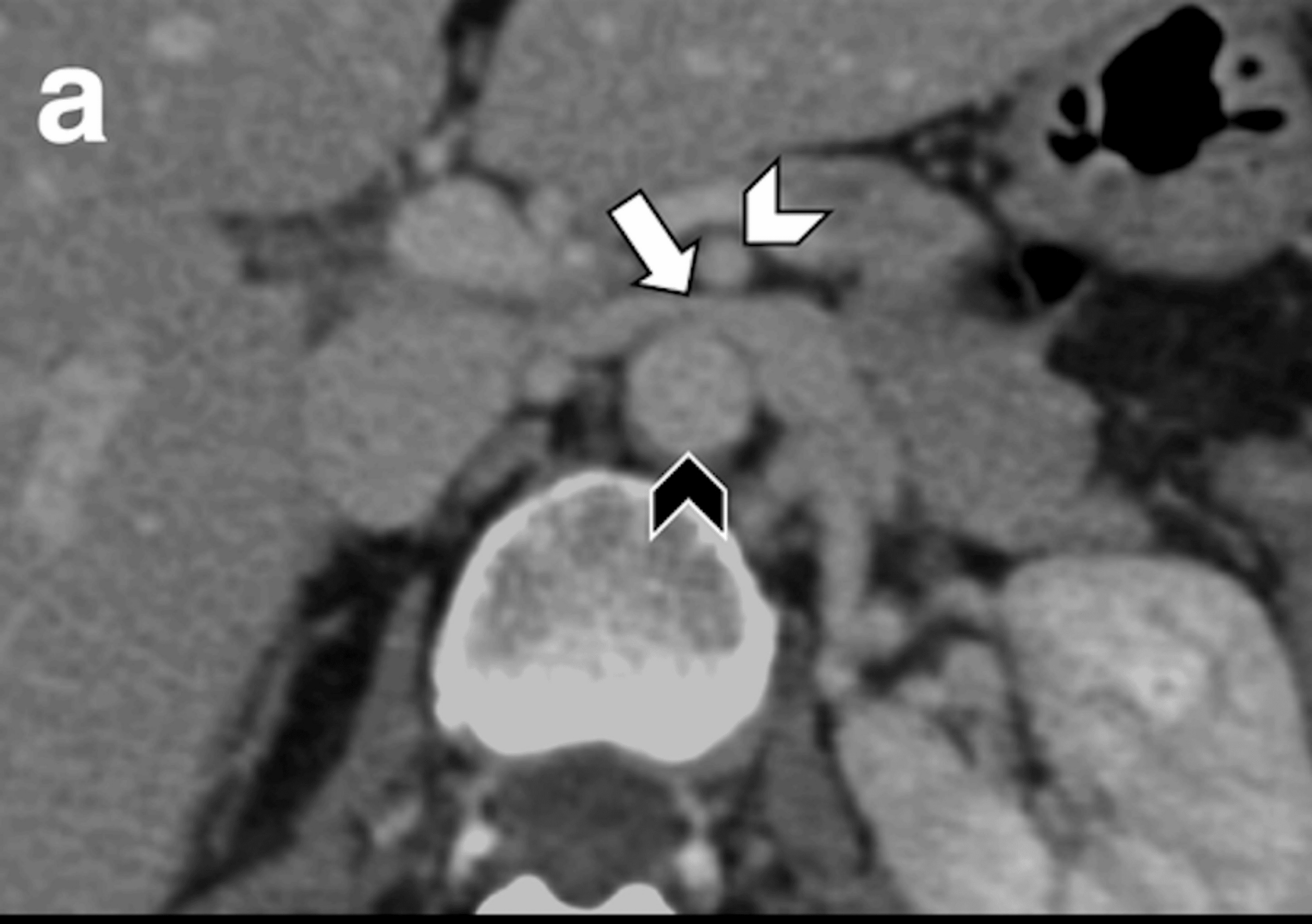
Axial view CT scan with contrast showing a “beak sign” (white arrow) indicating compression of the left renal vein between the aorta (black arrowhead) and the superior mesenteric artery (white arrowhead). The figure is taken from Gozzo et al. [1]. This article is licensed under a Creative Commons Attribution 4.0 International License, which permits use, sharing, adaptation, distribution, and reproduction in any medium or format, as long as you give appropriate credit to the original author(s) and the source (http://creativecommons.org/licenses/by/4.0/). CT: computed tomography.
